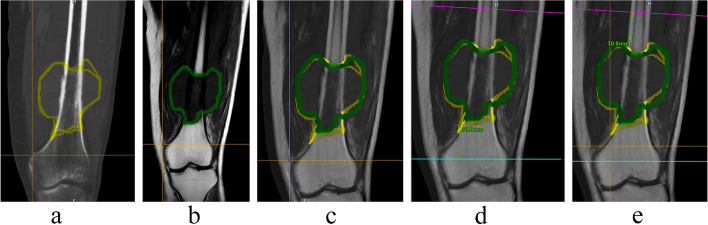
Preoperative planning on the navigation workstation. a CT and the tumor. b MRI and the tumor. c The fused image of CT and MRI with manually drawn tumor. d The designed margin for distal part with a plane on the navigation workstation. e The designed margin for proximal part with a plane
